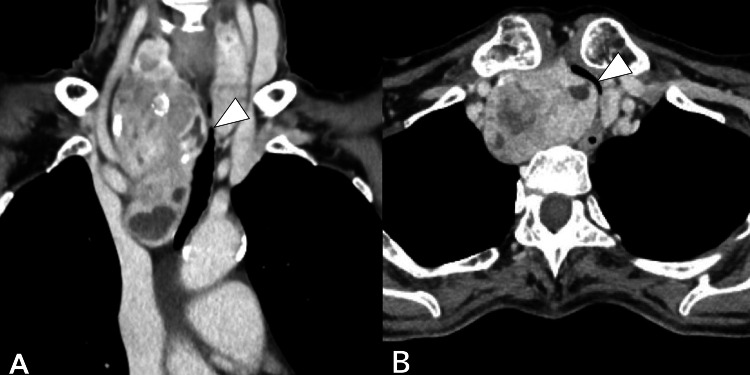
Preoperative CT Images Preoperative chest CT images with coronal view (A) and axial view (B) showing a massive right goiter descending from the right lobe of the thyroid gland to the middle mediastinum. Arrowheads indicate the narrowest parts of the trachea. CT: computed tomography
